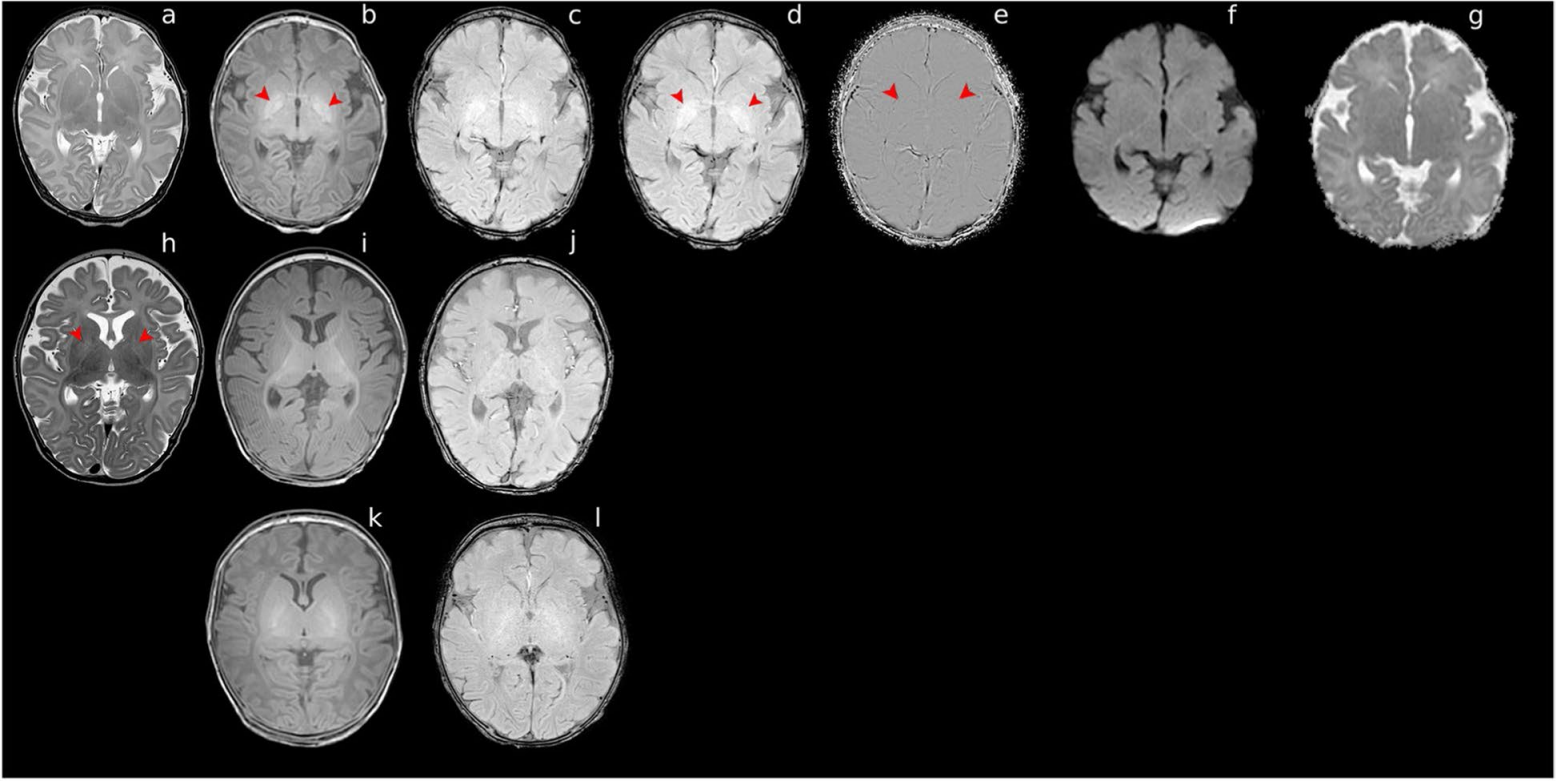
MR images in an 8-day-old term infant. Upper row: Axial T2 (TR 6282 ms, TE 120 ms, slice thickness 2 mm, no gap) shows no high signal intensity at globus pallidus level (a). Axial 3D T1(TR 9,38 ms, TE 4,6 ms, slice thickness 2 mm) shows a high signal in the basal ganglia (b). SWI (spoiled T1 enhanced with TR 31 ms, TE first 7,2 ms, slice thickness 2 mm) shows a high signal at the subthalamus (c) and globus pallidus level (d). The Phase image at the level of globus pallidus level shows no abnormal signal compatible with calcifications or blood products (e) Axial DWI, b 1000 and ADC map (TR 4998 ms, TE 102 ms, slice thickness 3 mm) shows no abnormal increased signal in basal ganglia (f, g). Middle row: At three months of age, the followup MR shows normalization of the signal on T1 and SWI (i and j) and a high T2 signal at the globus pallidal level (h). Lower row: Term neonate as control scanned in the first week of life. A normal signal at the basal ganglia on Axial 3D T1(TR 9,38 ms, TE 4,6 ms, slice thickness 2 mm) (k) and SWI (spoiled T1 enhanced with TR 31 ms, TE first 7,2 ms, slice thickness 2 mm) (l)
